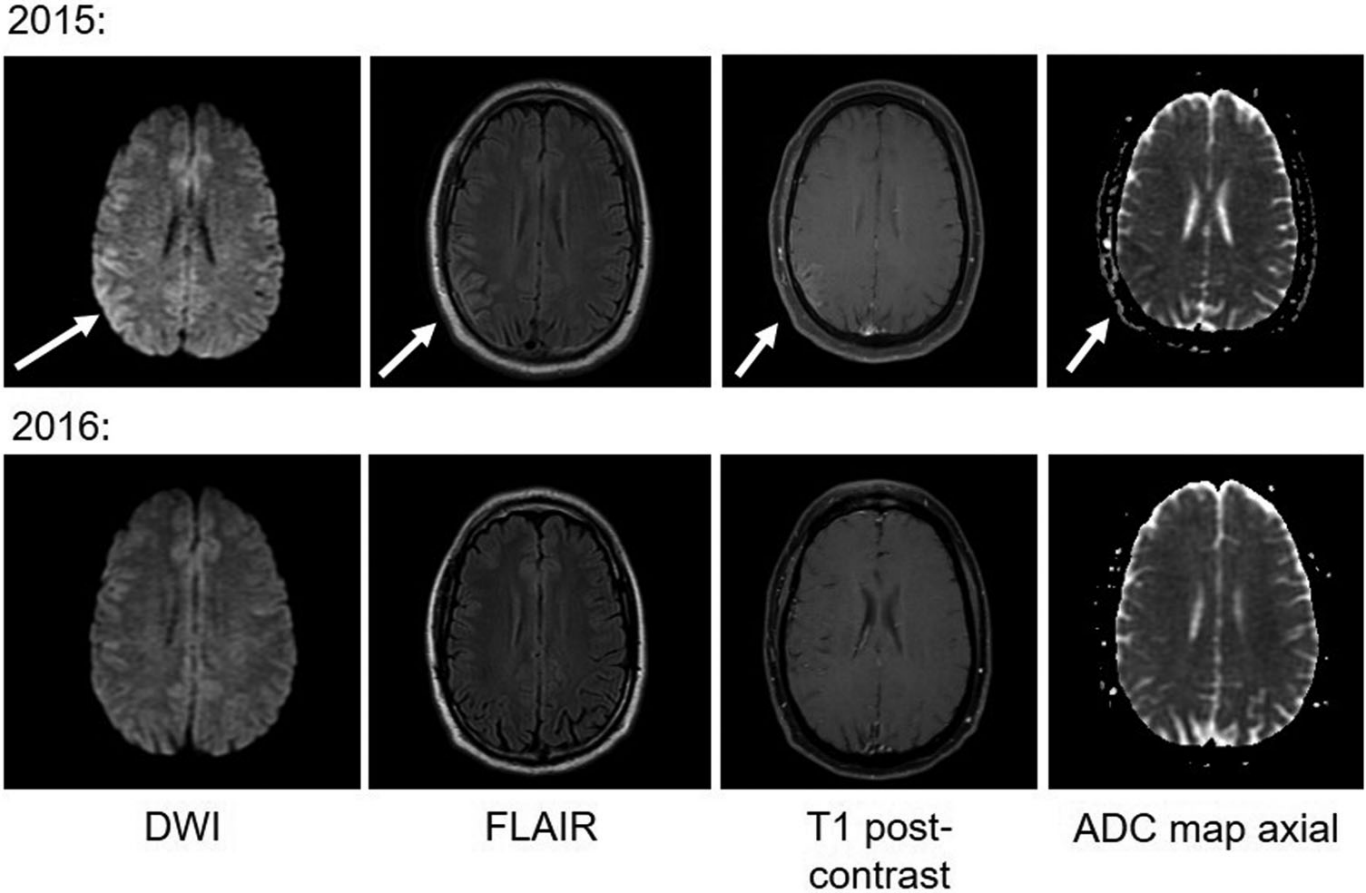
Brain MRI findings from Patient 5. Arrows highlight areas of abnormality in the right parietal cortex, which resolved over 14 months.
